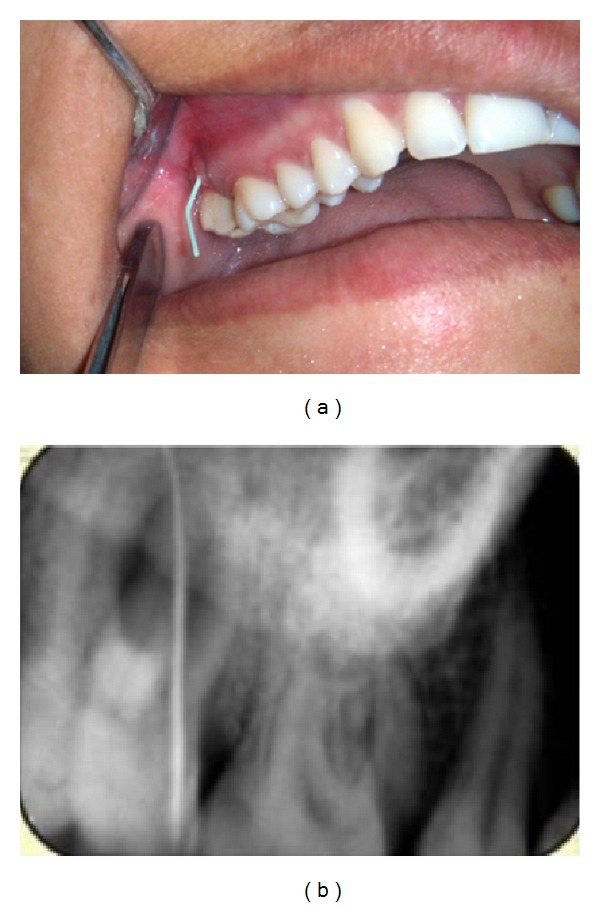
Clinical and radiographic tracing of sinus.
